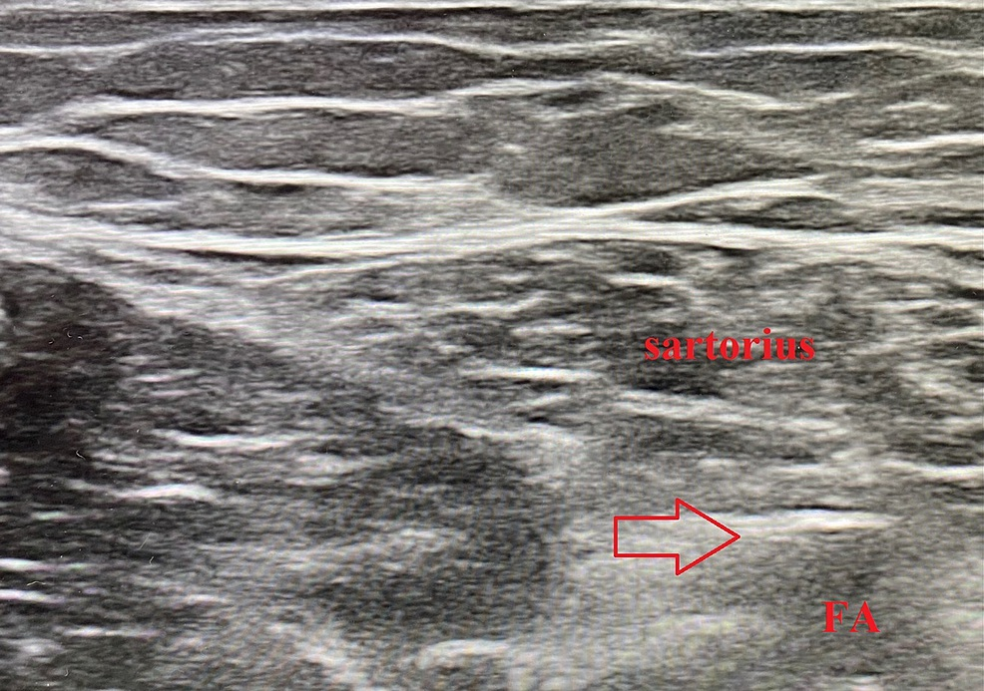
Ultrasound imaging of the adductor canal. Ultrasound was used to identify the sartorius muscle and the adductor canal. The adductor canal contains the femoral artery, femoral vein, and saphenous nerve. The needle was placed in the region near the red arrow which provided adequate stimulation of the saphenous nerve
